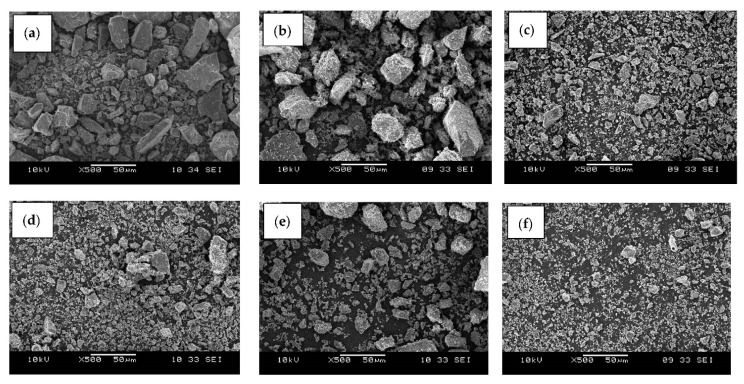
SEM images of dolomite that has been ball-milled for 500 rpm and ultrasonicated for 2 h with (a) 30% amplitude and 1 repetition (D_301X_), (b) 40% amplitude and 2 repetitions (D_402X_), (c) 40% amplitude and 3 repetitions (D_403X_), (d) 50% amplitude and 2 repetitions (D_502X_), (e) 50% amplitude and 3 repetitions (D_503X_), and (f) 50% amplitude and 10 repetitions (D_510X_).
